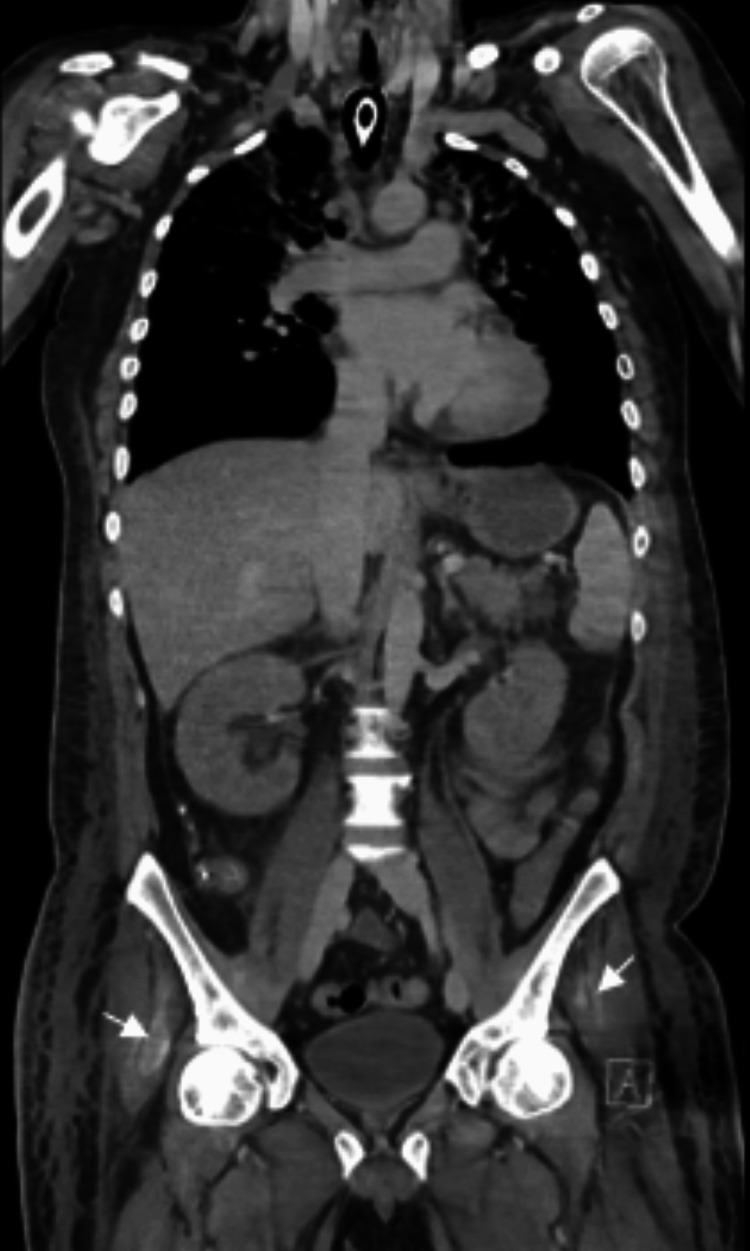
Calcium deposition in rhabdomyolysis-associated muscle injury Contrast-enhanced axial computed tomography of the abdomen and pelvis obtained on hospital day 26 demonstrates abnormal heterogeneous enhancement of the gluteal musculature (arrows).
